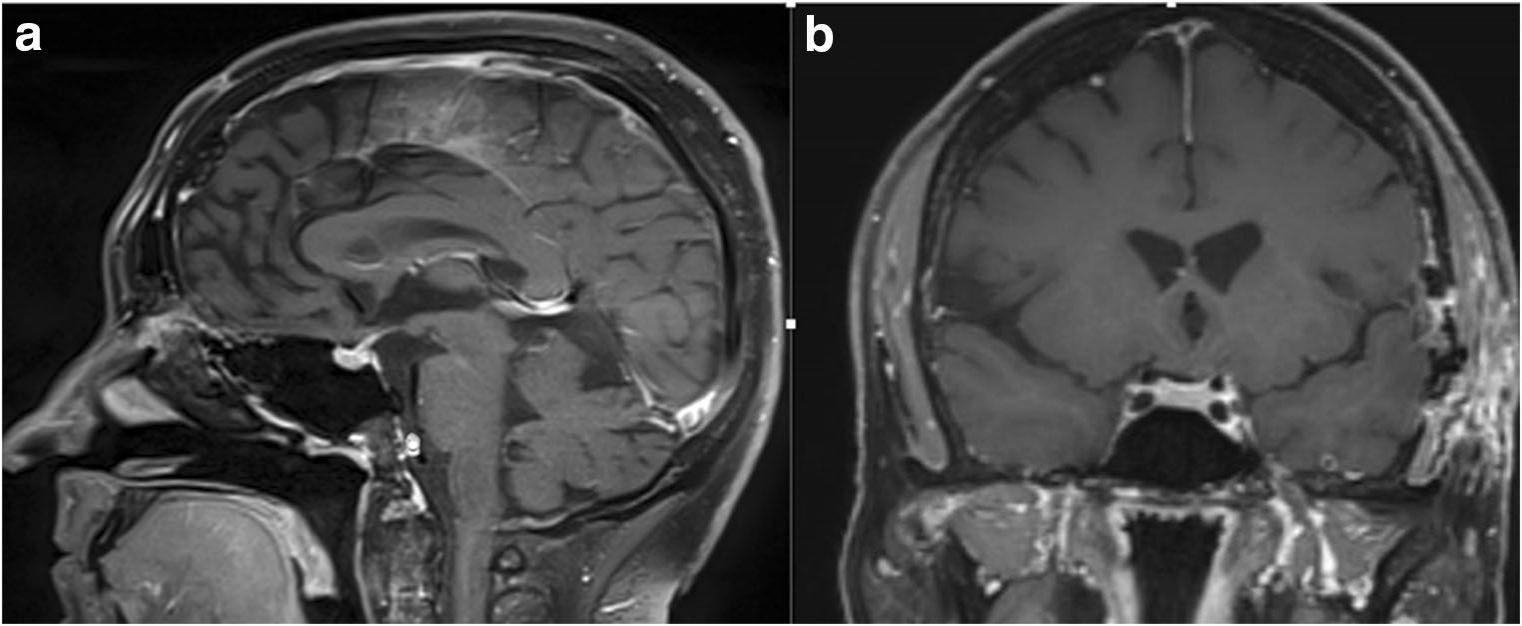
Postoperative sagittal (a) and coronal (b) MR images showing no residual tumor
